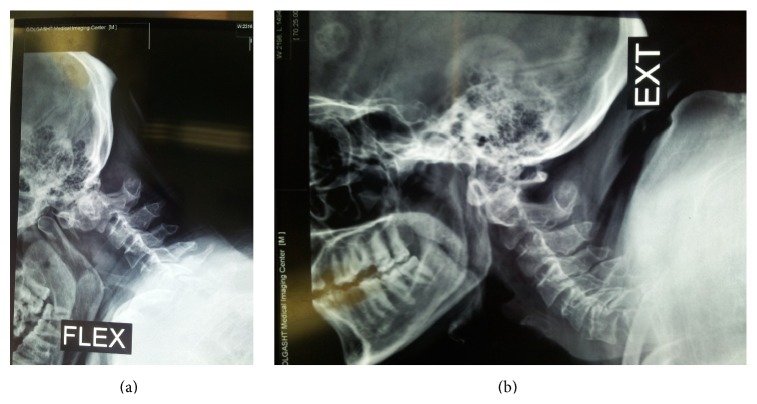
((a) and (b)) Dynamic lateral cervical radiographs showing atlantoaxial dislocation.
